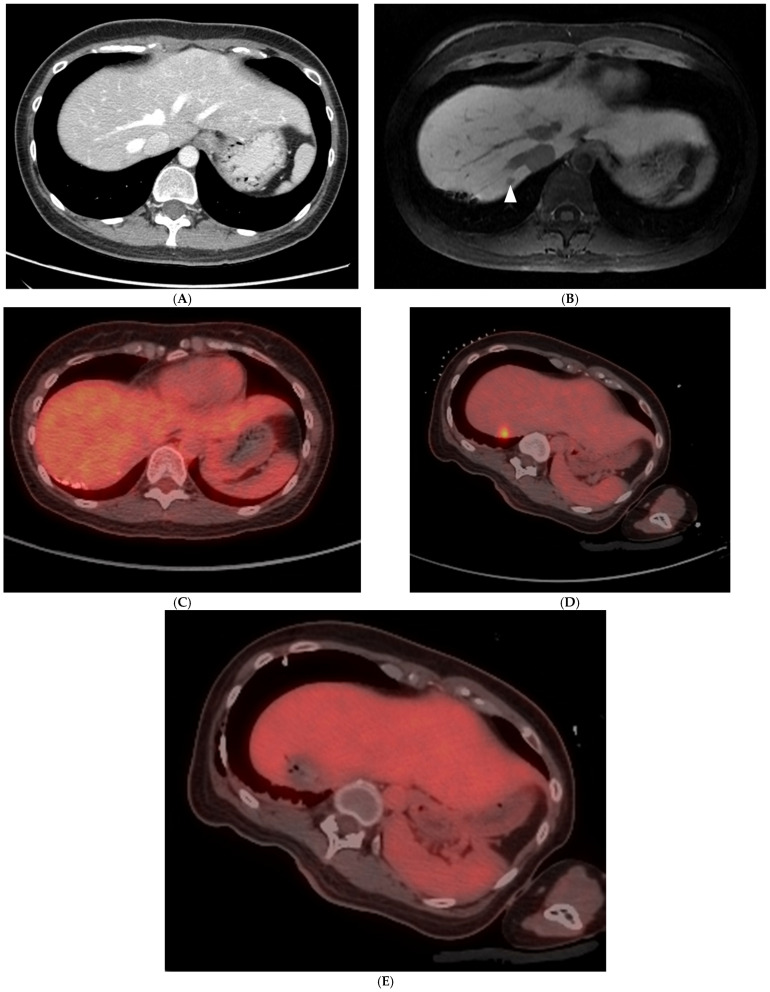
A 39-year-old woman with metastatic rectal cancer, post-liver resection, and hepatic arterial infusion pump placement, on FOLFIRI chemotherapy with solitary 0.7 cm segment 7 metastasis. The metastasis was subtle on the portal venous phase CT (A) and conspicuous on the hepatobiliary phase of the MRI ((B); white arrowhead). The tumor did not demonstrate increased focal FDG uptake on baseline PET/CT (C). Following a 4-week chemotherapy break, the patient presented to interventional radiology for percutaneous microwave ablation. The target demonstrated FDG-avidity after discontinuation of chemotherapy (D), permitting PET-guided microwave ablation using the split-dose technique. Immediate post-ablation PET/CT confirms a photopenic defect at the tumor site (E).
